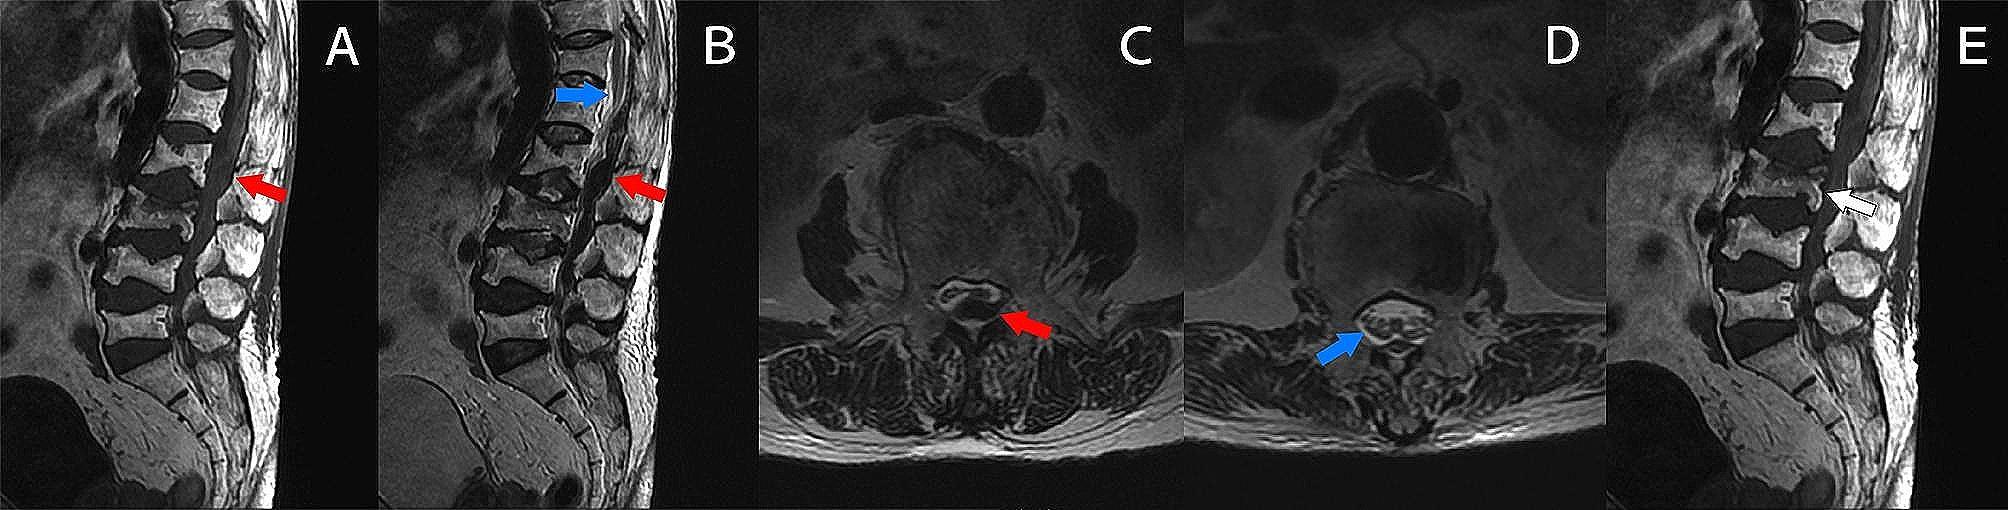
Initial MRI performed five days after symptoms onset revealed a dorsal SEH at the level of L1-L4 with compression of the thecal sac (red arrow) (A-C) but not the conus medullaris (blue arrow)(D). The SEH displayed a mixed signal with isointense and slightly hypointense to the spinal cord on T1WI sagittal image(A) and hypointense on T2WI sagittal image (B) and T2WI axial image showing(C). Additionally, the images revealed a recent L3 vertebral compression fracture (white arrow) (E)
